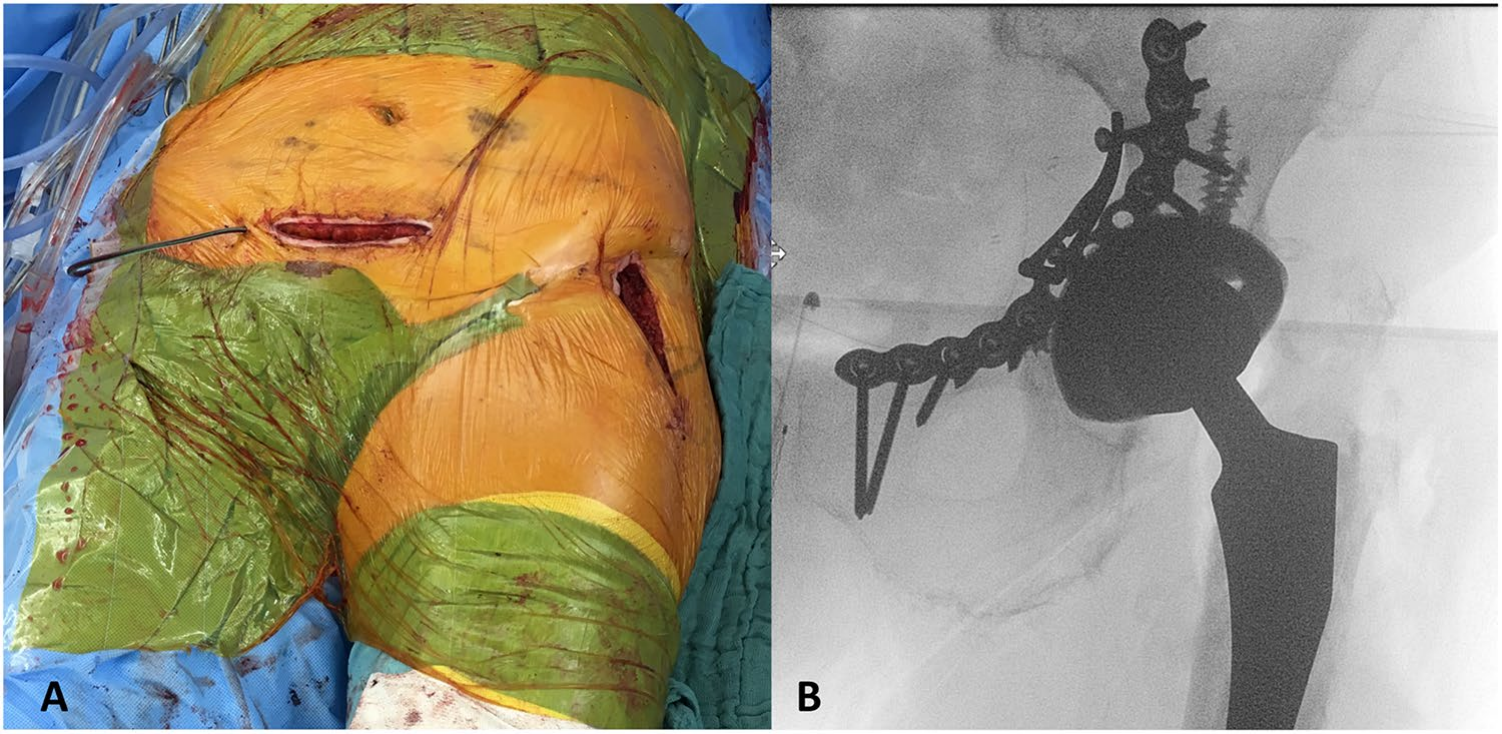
a Intraoperative picture of the AIP and DAA approaches combined, and b corresponding final intraoperative radiograph after finished acute total hip arthroplasty
